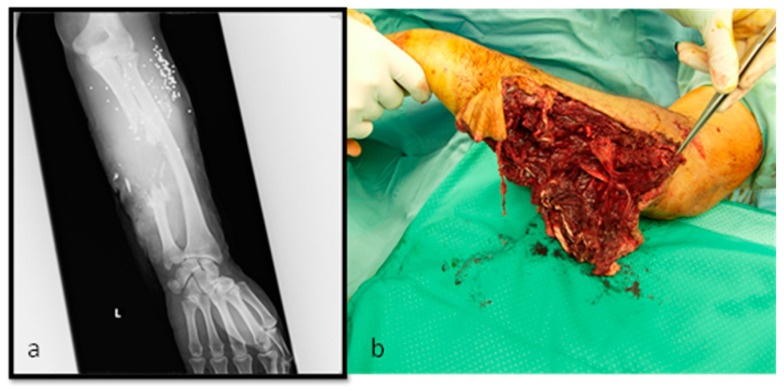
M.E. 50 years old, (a) initial x-ray and (b) clinical status, classified as IIIC G/A score.
